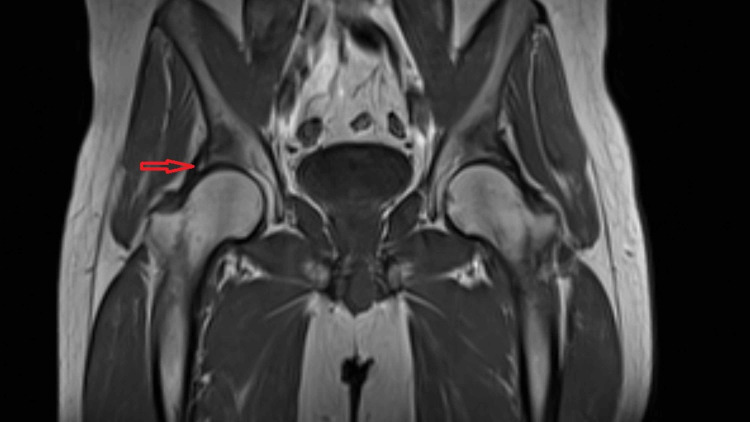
Coronal slice of T1-weighted MRI showing fracture of acetabulum (red arrow)
